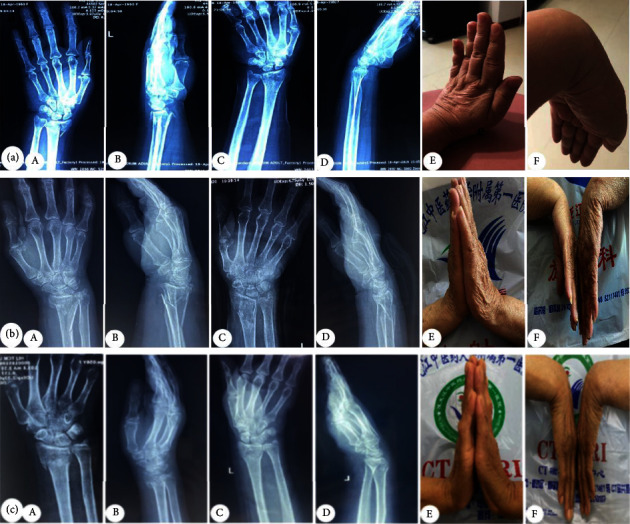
Anteroposterior and lateral radiographs of the Colles fracture before and after reduction show that volar inclination and ulnar deviation returned to normal, and wrist deformity disappeared. Using the integrated retainer pad splint of the distal radius, the radial height, volar inclination, and ulnar deviation of the wrist were maintained easily and effectively. (a) Typical case 1; (b) typical case 2; (c) typical case 3; (A) anteroposterior radiograph before reduction; (B) lateral radiograph before reduction; (C) anteroposterior radiograph after reduction; (D) lateral radiograph after reduction; (E) wrist dorsiflexion function at the 8th week follow-up; (F) wrist palmar flexion function at the 8th week follow-up.
